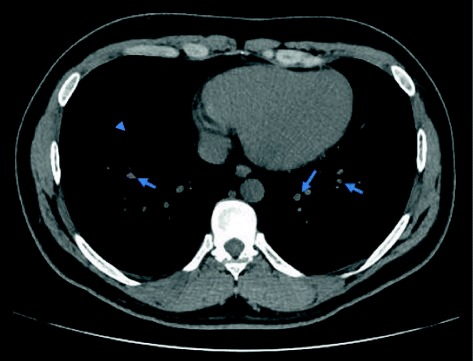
Plain HRCT chest depicting parenchymal lesion with calcification
